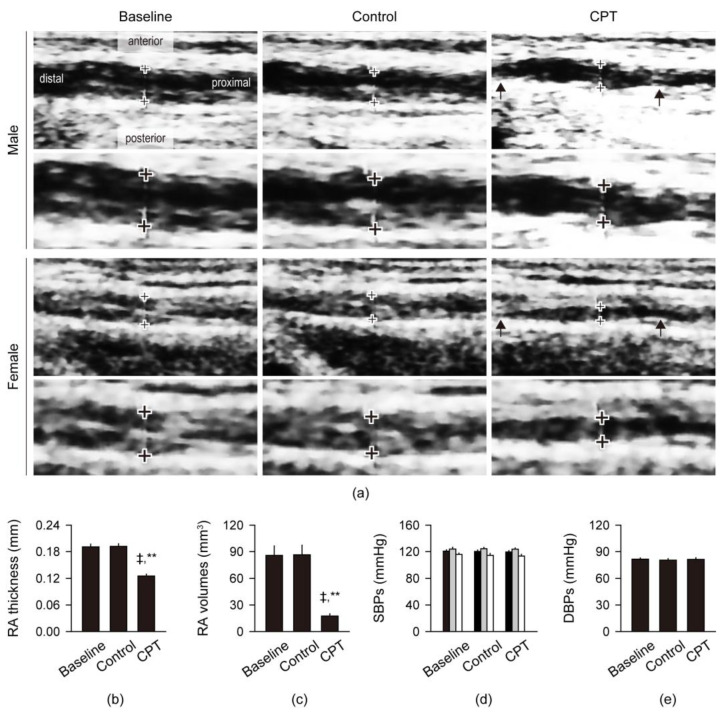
Ultrasonography of the radial artery and blood pressure. (a) Representative images of the radial artery (RA). The pulse diagnostic regions (+) were high-magnified in lower in the males and females. Arrows indicate evident vasoconstriction of the RA; (b,c) RA thickness and volumes in the pulse diagnostic region; (d,e) Systolic/diastolic blood pressures (SBPs/DBPs). Black, gray, and white bars indicate the values in the total subjects, males and females. Values are represented as the means ± SEMs. ‡ and : *p* < 0.01 versus the baseline and control trial, respectively, by the Paired-sample *t*-tests.
